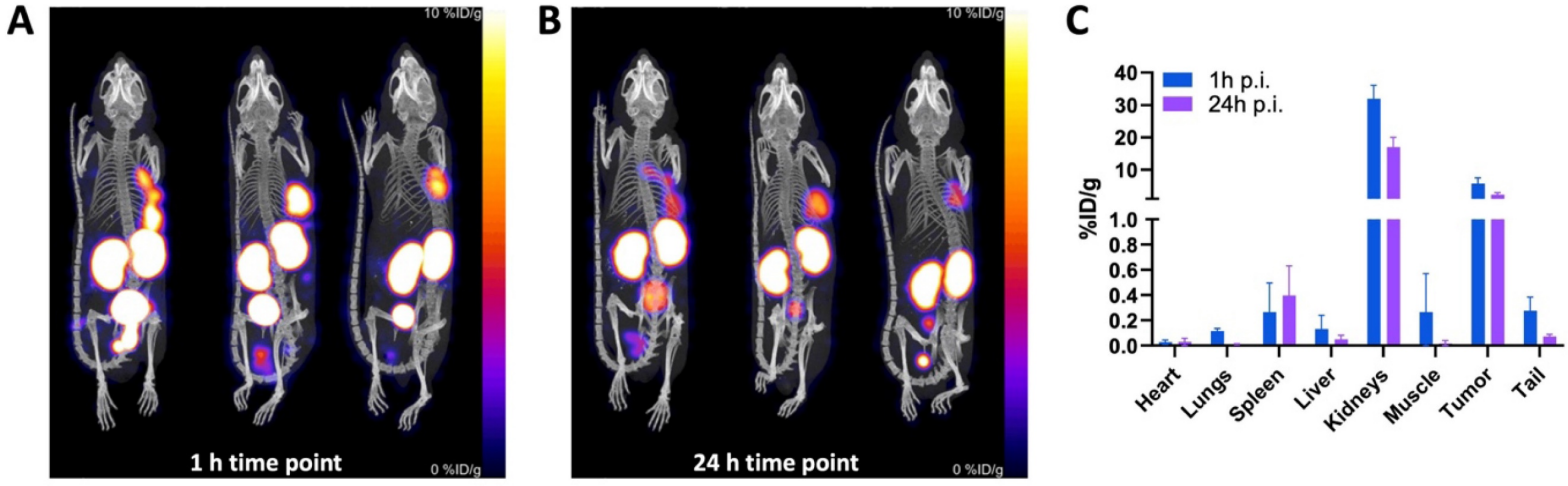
SPECT/CT imaging of [^111^In]In-BCY18469 in a PC-3 xenograft model. A) Maximum intensity projection at 1 h and B) 24 h p.i. of 230 pmol (5.3 ± 0.2 MBq) of [^111^In]In-BCY18469. C) Quantified data (%ID/g) of [^111^In]In-BCY18469 SPECT imaging.
